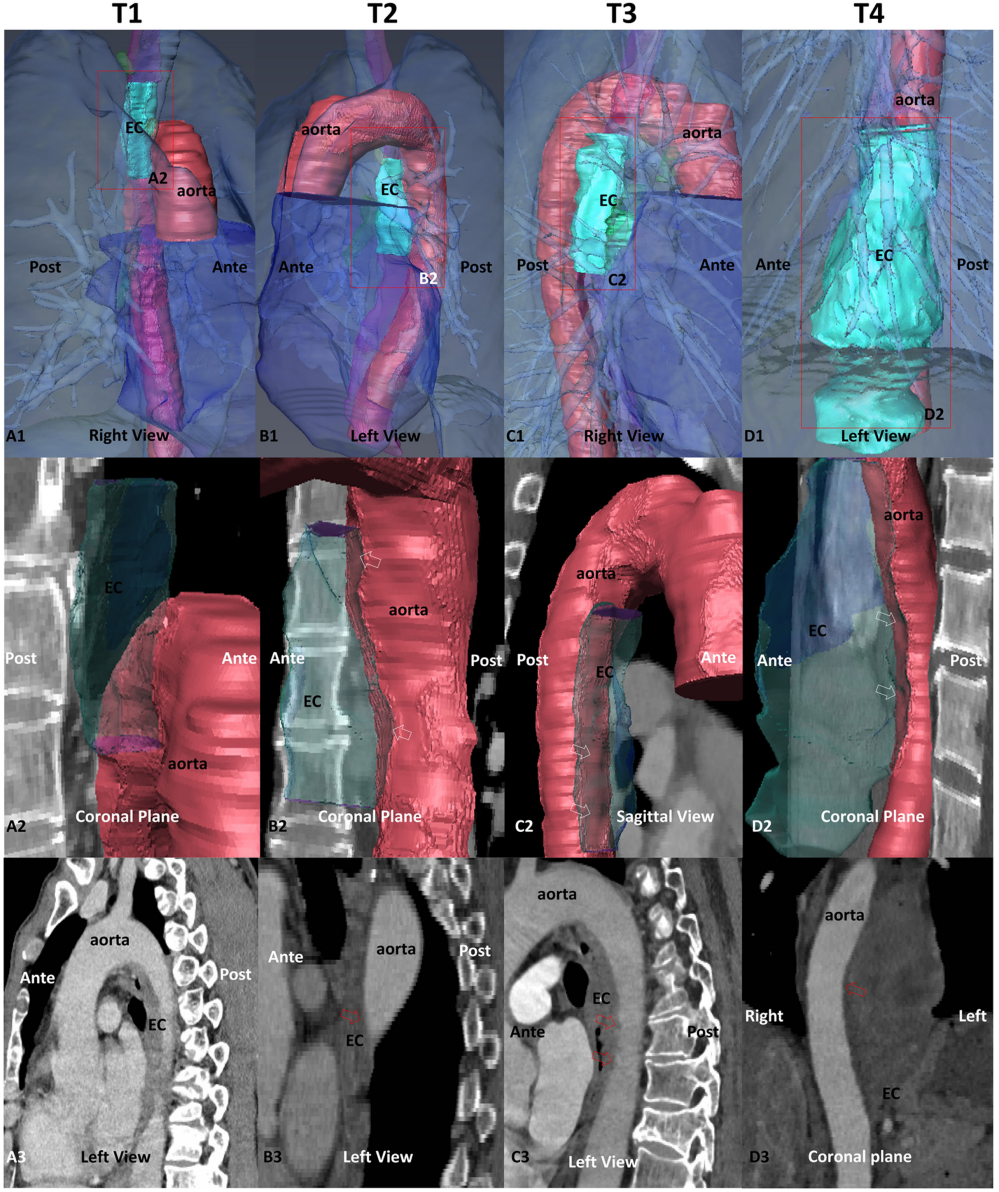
Relationship between tumour and aorta in the 3D reconstructed model. A1–D1 are 3D reconstructions of EC and its adjacent structures; A2–D2 are 3D reconstructed tumours (with transparency treatment) with superimposed sagittal CTA images of the aorta, allowing easier assessment of the relative position of the tumour in relation to the aorta, and also affording clear visualisation of aortic vessel wall compression by the tumour; A3–D3 are sagittal and coronal CTA images of the tumour region. E, normal oesophagus, EC, oesophageal cancer.
